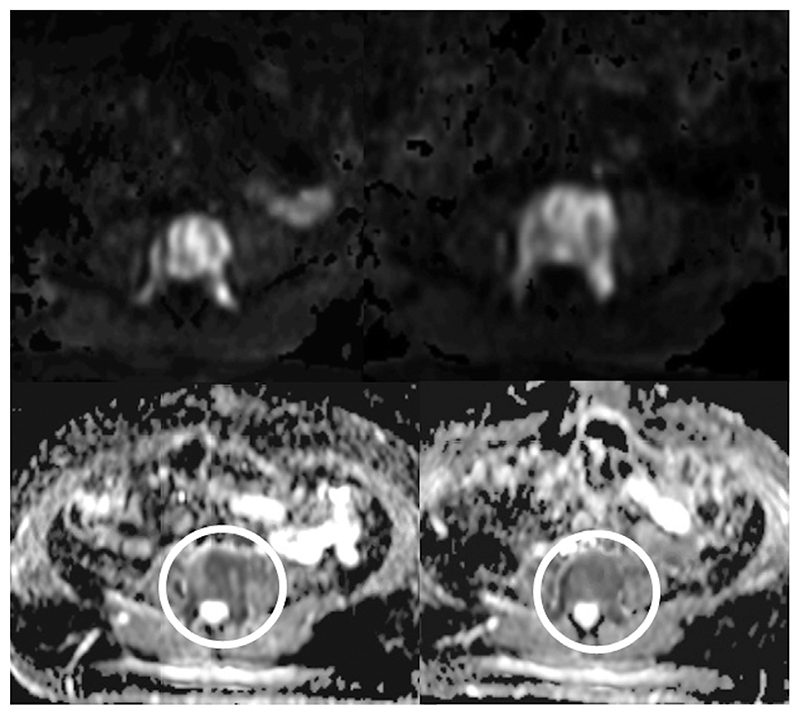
The same patient as Figure. 5. (a) T1-weighted (left) and T2-weighted (right) sagittal MRI of the spine showing low signal in L4 due to metastatic marrow infiltration (arrows). (b) Top row - axial b900 DWI at baseline and 8 weeks after therapy showing high signal in L4. The ADC map (bottom row) shows evidence of restricted diffusion in the vertebra (circles). The ADC increased from 900 to 1108 mm^2^/s in keeping with treatment response.
